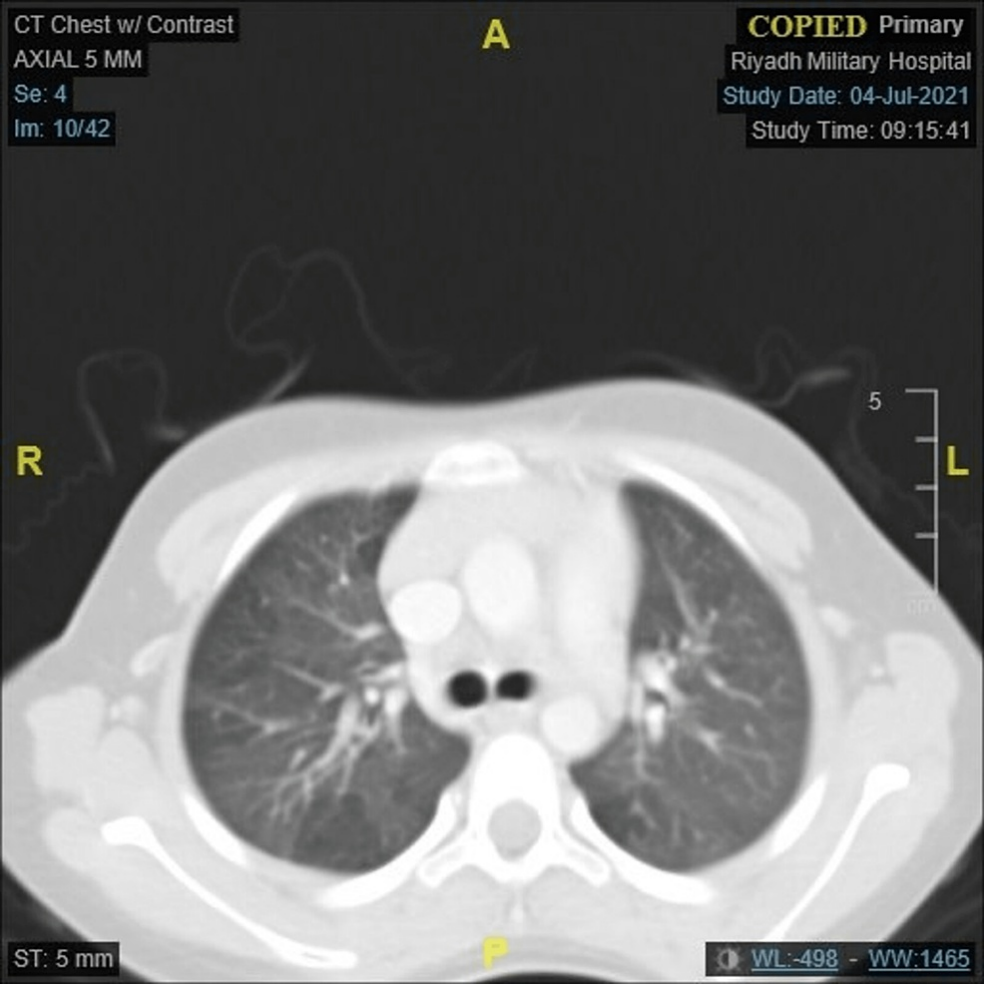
A CT scan showing the axial lung window, diffuse mosaic, ground-glass attenuation of the lungs suggestive of regional air trapping with no evidence of bronchiectasis
